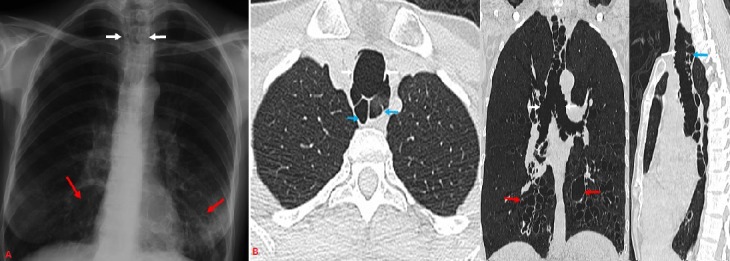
A) a chest X-ray showing an enlarged tracheal diameter (white arrows), with multiple cysts in the lower lobes (red arrows); B) computed tomography scan of the chest showing a dilatation of both the trachea and the two main bronchi (white arrows) with multiple diverticulae (blue arrows). It also revealed bilateral cystic bronchiectasis involving both lower lung zones (red arrows)
